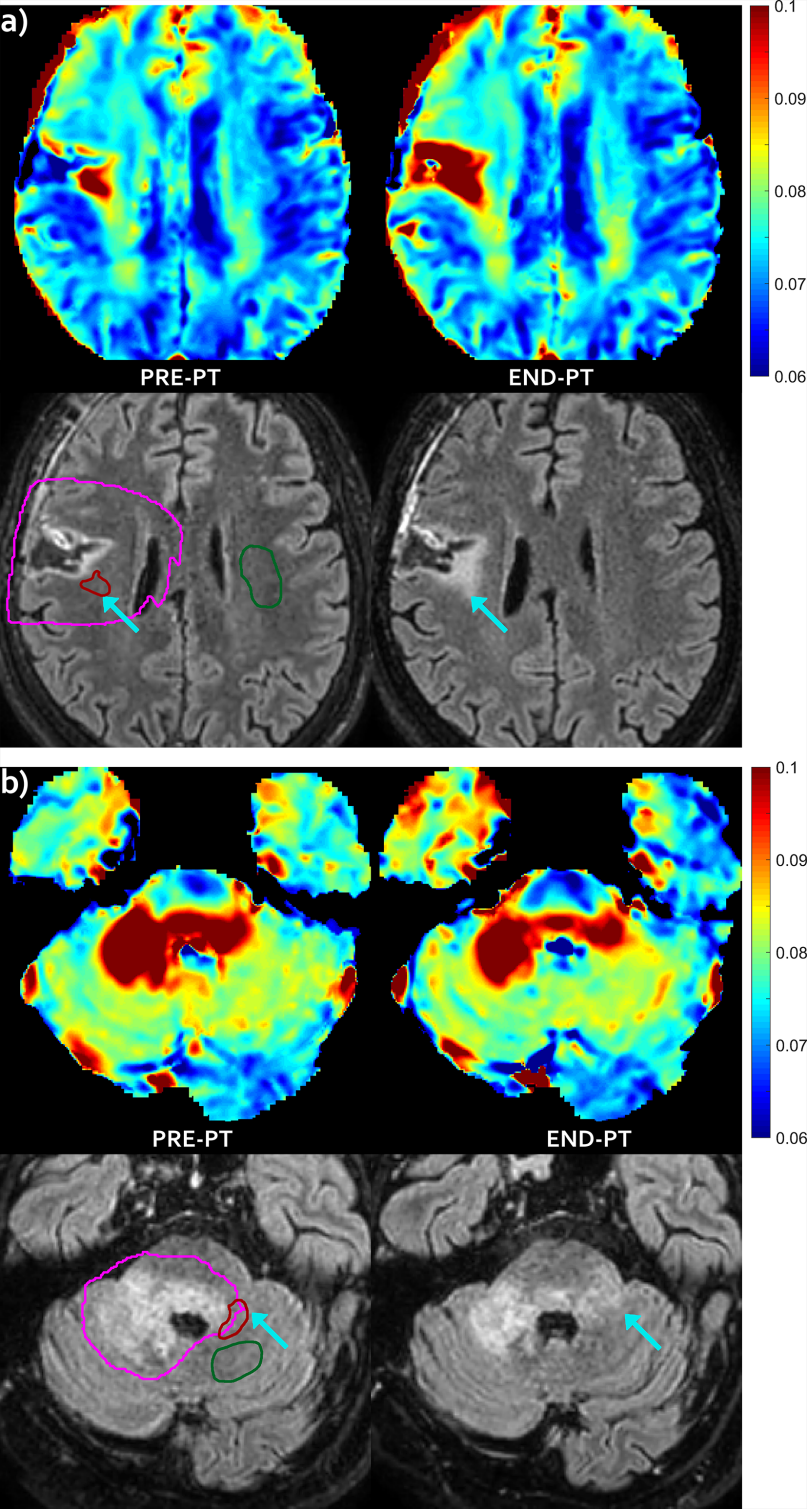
FLAIR increase pattern—Evolution pattern observed in patient #3 (A) and in patient #6 (B) as highlighted by the arrows. The mismatch, i.e. the abnormal T2 area that extended outside hyper-intense FLAIR area before proton irradiation (left) evolved into a new area of FLAIR hyper-intensity at end-PT (right), that was diagnosed as radiation induced damage. T2 intra/extracellular water maps (top) and FLAIR images (bottom) are shown. Colored bar is shown in seconds. On pre-PT FLAIR image the contours of the CTV (violet line), of the mismatch ROI (red line) and of the contralateral healthy ROI (green line) are shown.
